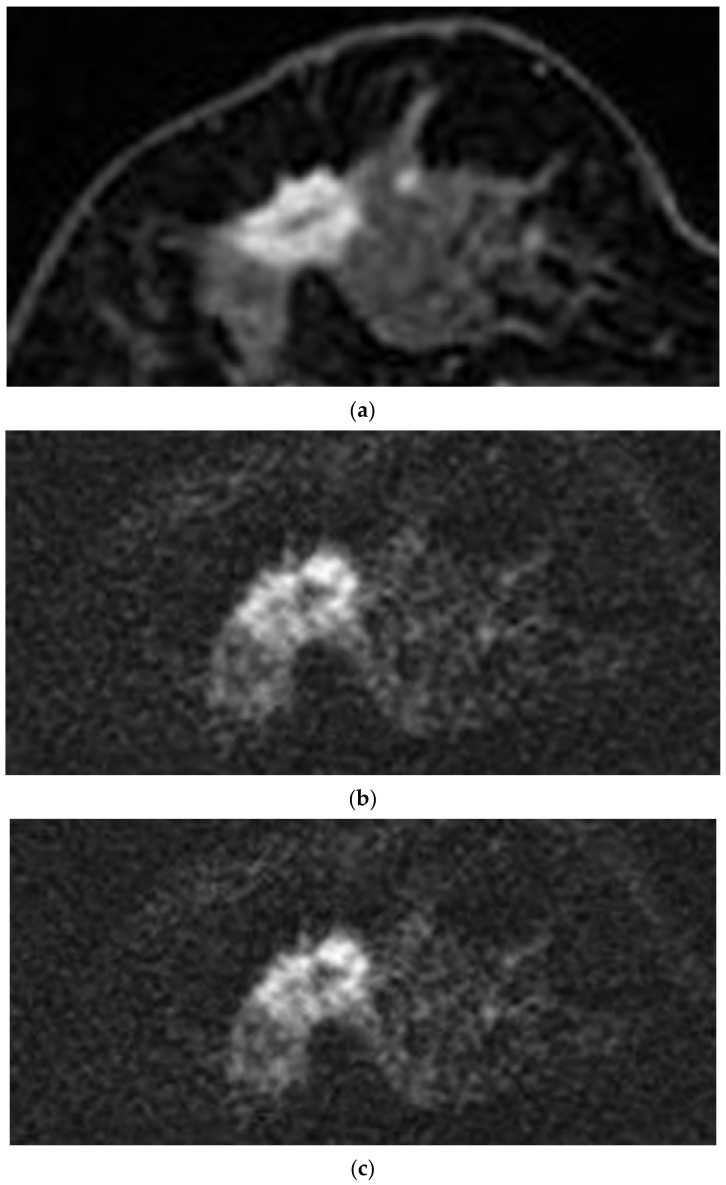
A 47-year old woman who was diagnosed invasive ductal carcinoma in her right breast. Axial contrast-enhanced T1-weighted fat-suppressed MRI (a) reveals a 16 × 12 mm-sized enhancing mass with irregular shape, spiculated margin, and rim, and marked enhancement is noted in the right breast at the 9 o’clock position. rFOVA-1000 ^a^ (b) and rFOVS-1000 ^b^ (c) also show index tumor well and had excellent depiction of shape irregularity and margin spiculation. ^a^ rFOVA-1000: acquired reduced field-of-view diffusion-weighted images with *b*-value 1000 s/mm^2^. ^b^ rFOVS-1000: synthetic reduced field-of-view diffusion-weighted images with *b*-value 1000 s/mm^2^.
